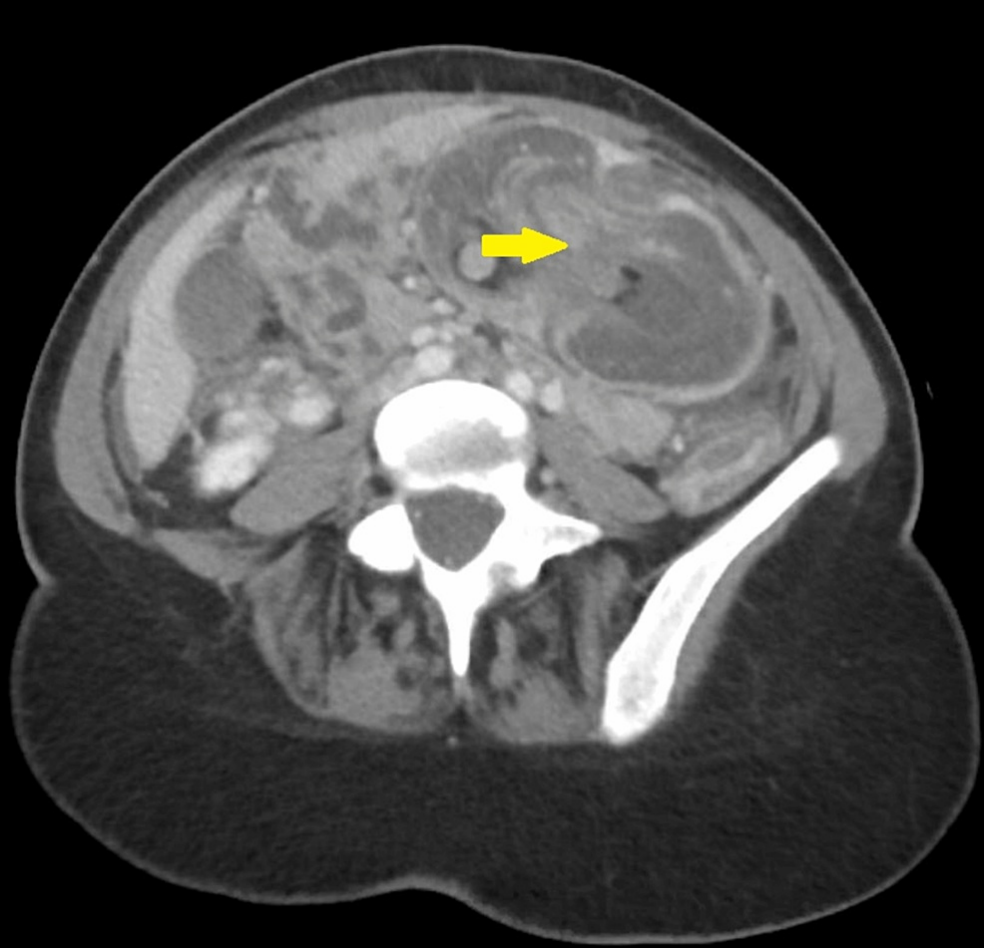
Transverse colo-colonic intussusception in the axial cut of abdominal computed tomography scan (yellow arrow)
